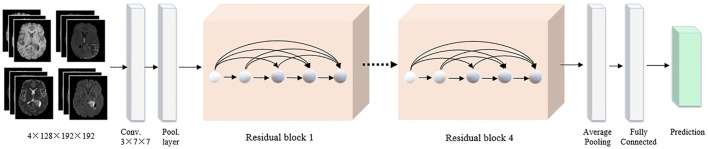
The detailed 3D CNN network. The four MRI modalities are integrated as the network input. All images are cropped to a fixed size of 128 × 192 × 192 pixels. The backbone adopts the 3D ResNet, followed by a global average pooling and a fully connected layer to classify the brain tumor.
